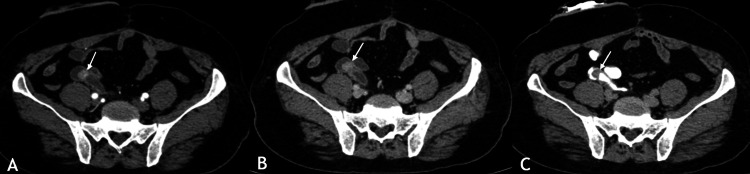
Triple-phase axial contrast-enhanced CT images demonstrate a distal left ureteric lesion at the uretero-enteric anastomosis (white arrows) Arterial phase (A) shows an avidly enhancing soft tissue lesion (white arrow) at the distal left ureter. Venous phase (B) reveals persistent enhancement of the lesion with improved contrast between the mass and adjacent conduit loops. The delayed excretory phase (C) shows continued enhancement of the lesion along with upstream mild left-sided hydroureteronephrosis, indicating partial functional obstruction. No regional lymphadenopathy or adjacent fat stranding was observed across phases.
